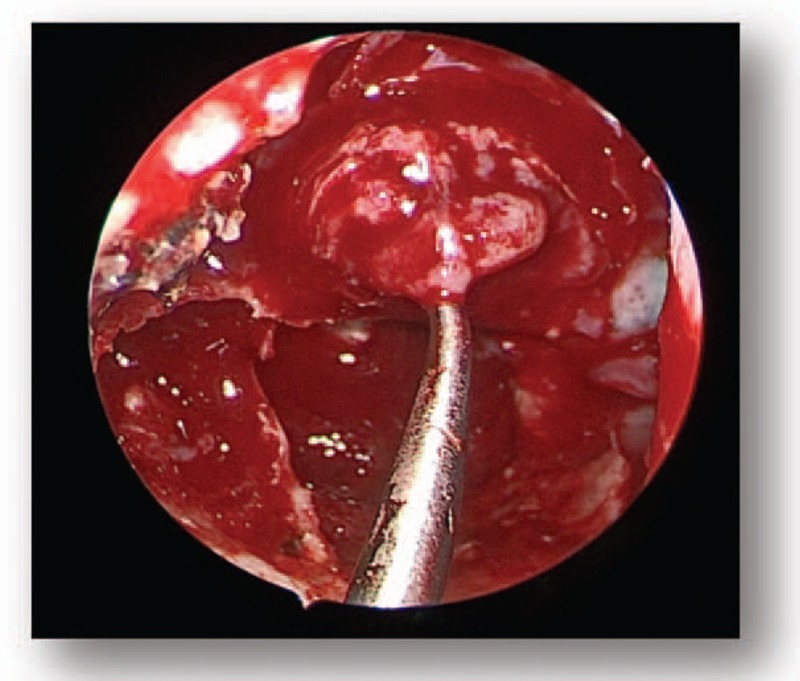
Preoperative MRI (D). MRI = magnetic resonance imaging.
